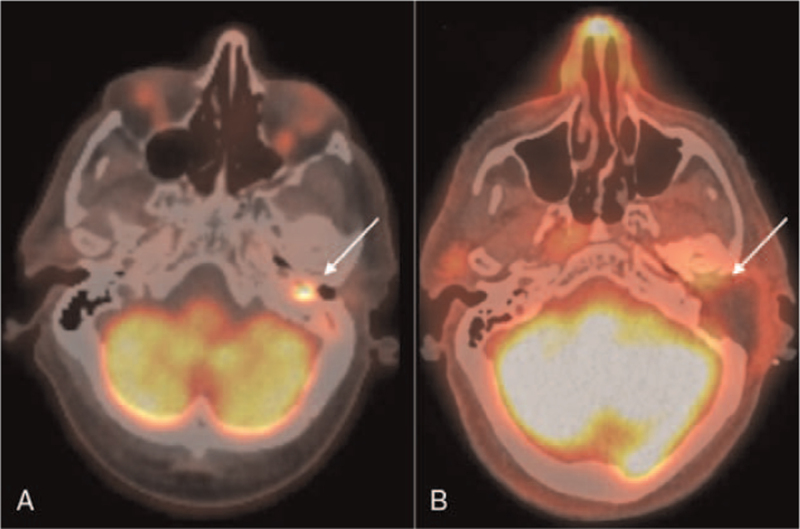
A*, Positron emission tomography/computed tomography with 2-[fluorine-18]-fluoro-2-deoxy-D-glucose (FDG-PET-CT) demonstrated intense FDG-uptake at the left middle ear cavity indicative for a malignant tumor. Only moderate FDG uptake was seen at the level of the external auditory canal and the mastoid bone. *B*, One month after surgery a FDG-PET-CT follow-up scan revealed minimal soft tissue induration at the petrosectomy site and mild FDG uptake with no evidence of residual tumor.
